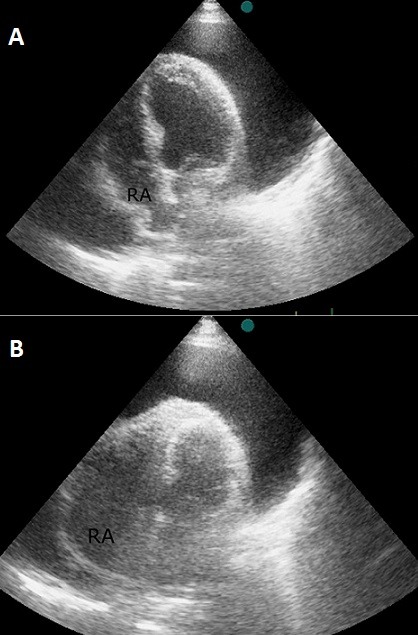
Transthoracic echocardiography (apical 4-chamber View) Large pericardial effusion with right atrial diastolic collapse (A) which missing at systole (B). Note the absence of right ventricular collapse. RA: right atrium
